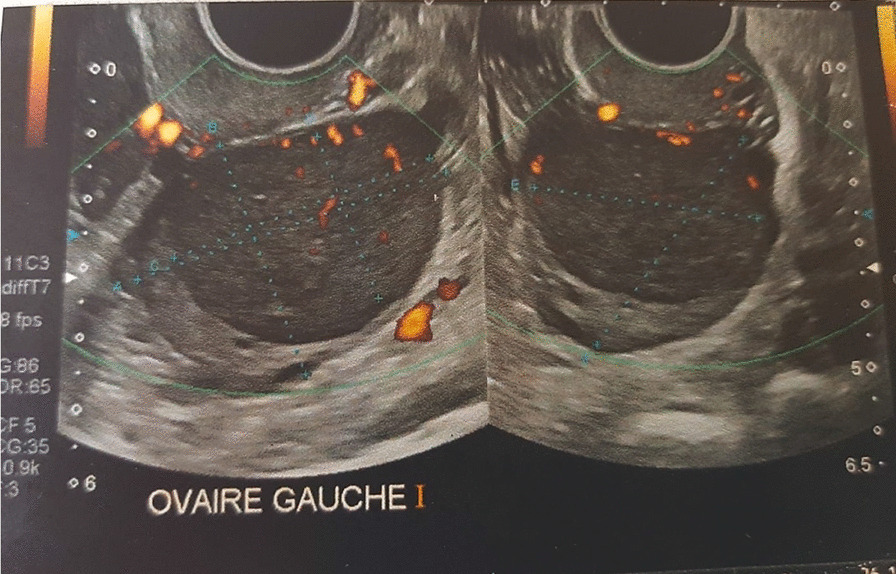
Ultrasound image showing an organic left ovarian mass measuring 39 mm
